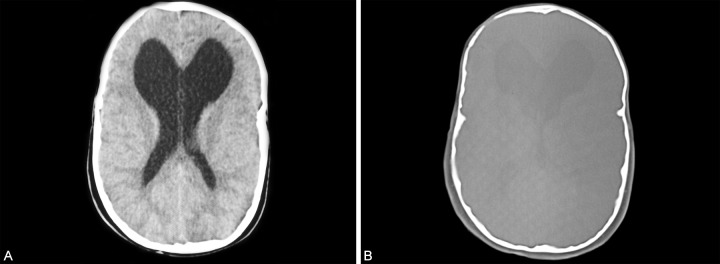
Moderate degree of hydrocephalus, diffuse indentation of inner table of skull seen in the CT scan
